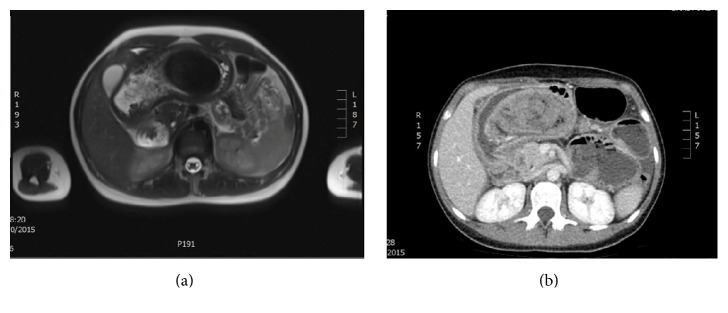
(a) MRI demonstrating two well-defined T2 hypointense and T1 isointense filling defect mass lesions within the stomach, extending into the proximal duodenum. The larger of these within the body of stomach measures approximately 100 × 50 mm (appears pedunculated) and the smaller of these in the gastric fundus measures 40 mm. (b) Preoperative CT confirms the presence of the mass in the patient's stomach.
